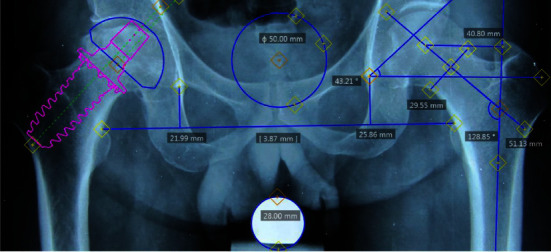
Preoperative planning on X-ray based on 2D software.
